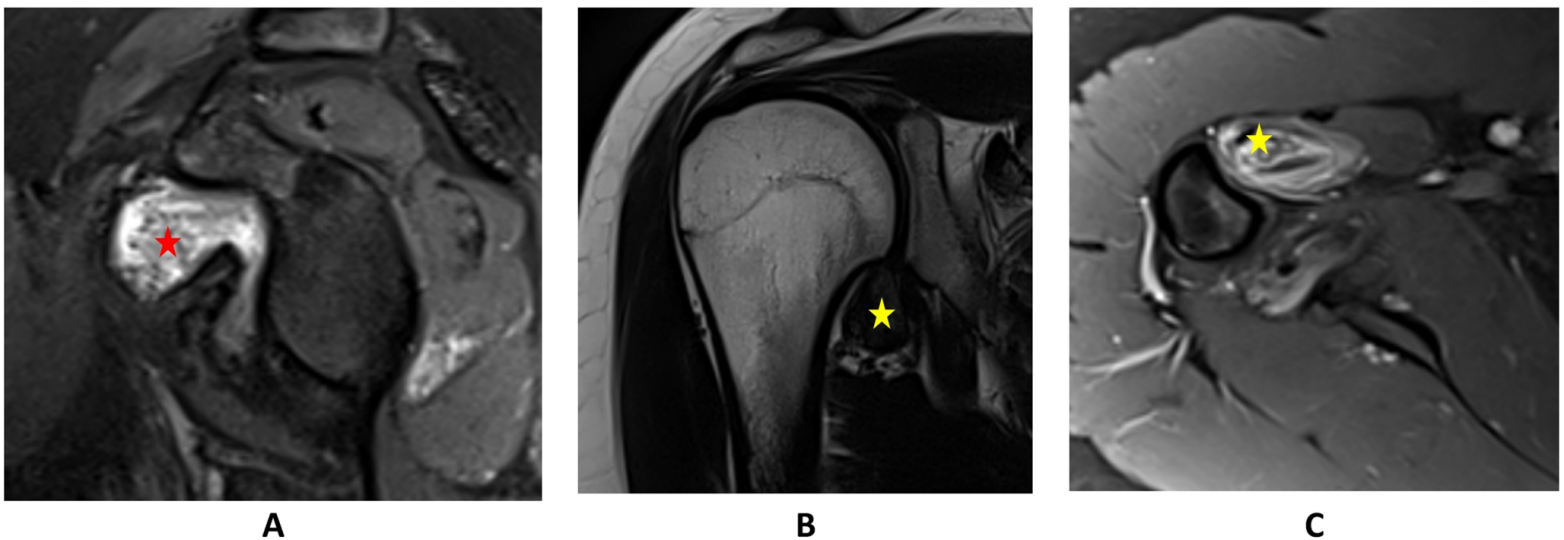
MRI scans of TGCT of the shoulder in patient 3. A: PDFS sagittal of the right shoulder: red star marks the mass below the coracoid. B: T2 coronal of the right shoulder: yellow star marks the mass in the lower recess. C: T1FS axial view of the right shoulder: yellow star marks the mass in the lower recess
